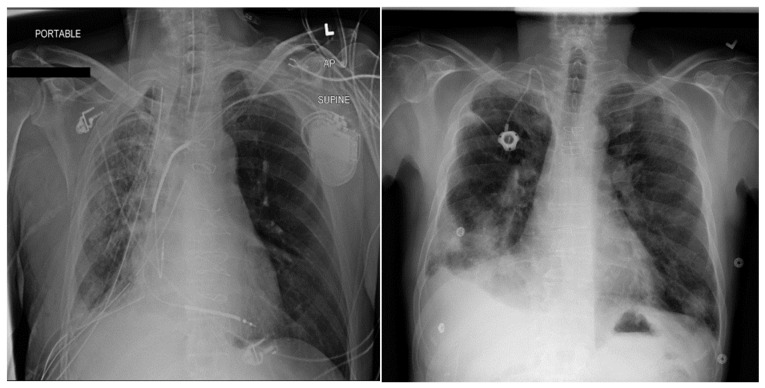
Sample Chest X-ray Images in the Dataset.
